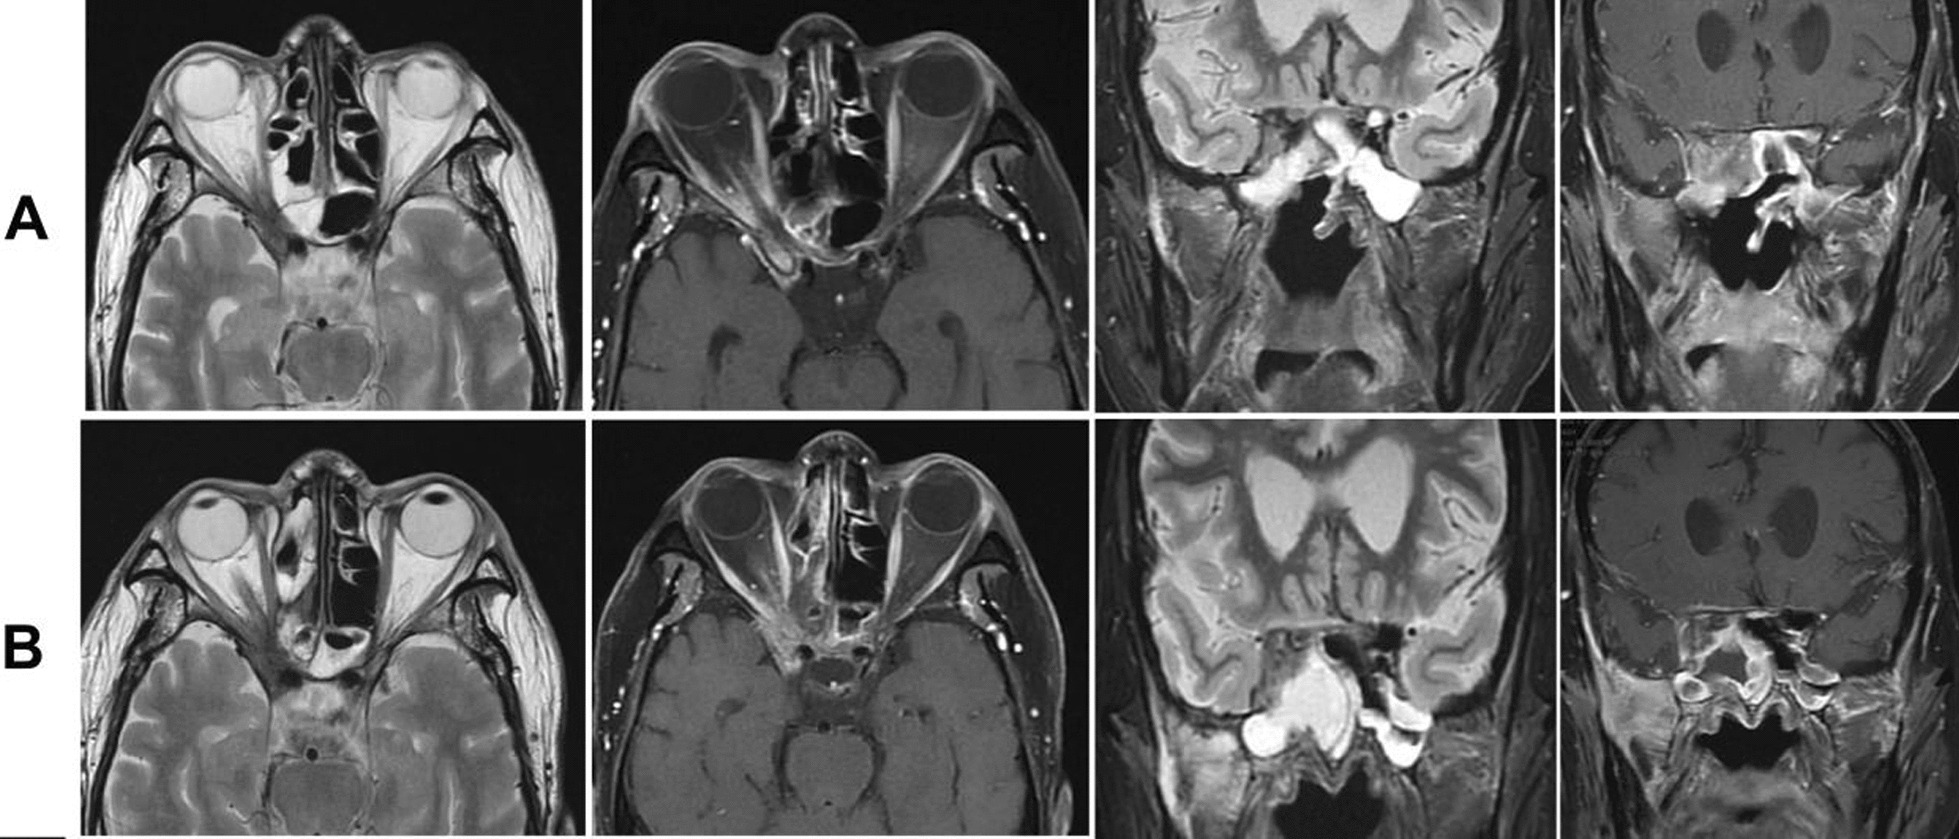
Comparison of magnetic resonance images of the sinuses before and after treatment: A 3 months after treatment; B before treatment
